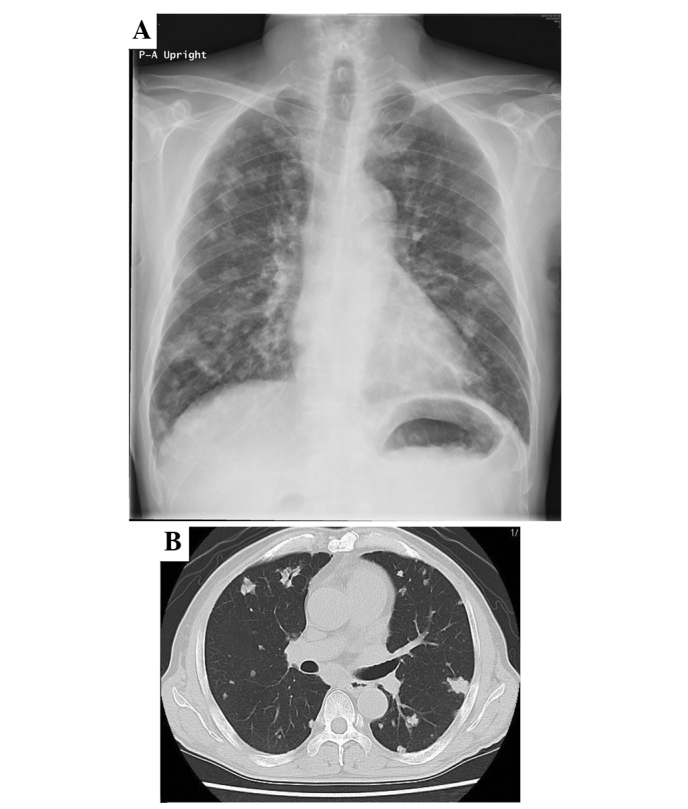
(A) Plain film and (B) CT of the lung at the last follow-up showing reduction in number and size of masses.
